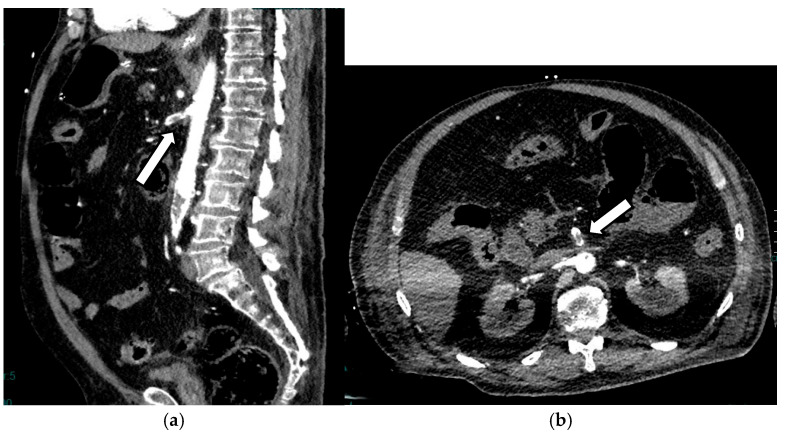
AAMI in a 78-year-old man: sagittal (a) and axial (b) contrast-enhanced CT scans show thrombotic occlusion at the emergence of the SMA (arrows).
